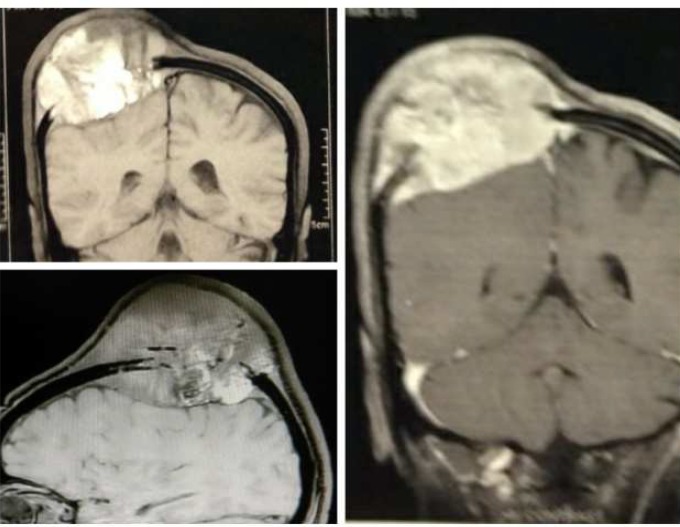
The coronal T1, sagittal FLAIR, and coronal Gd-enhancement T1 image sequences of brain magnetic resonance imaging (MRI)
